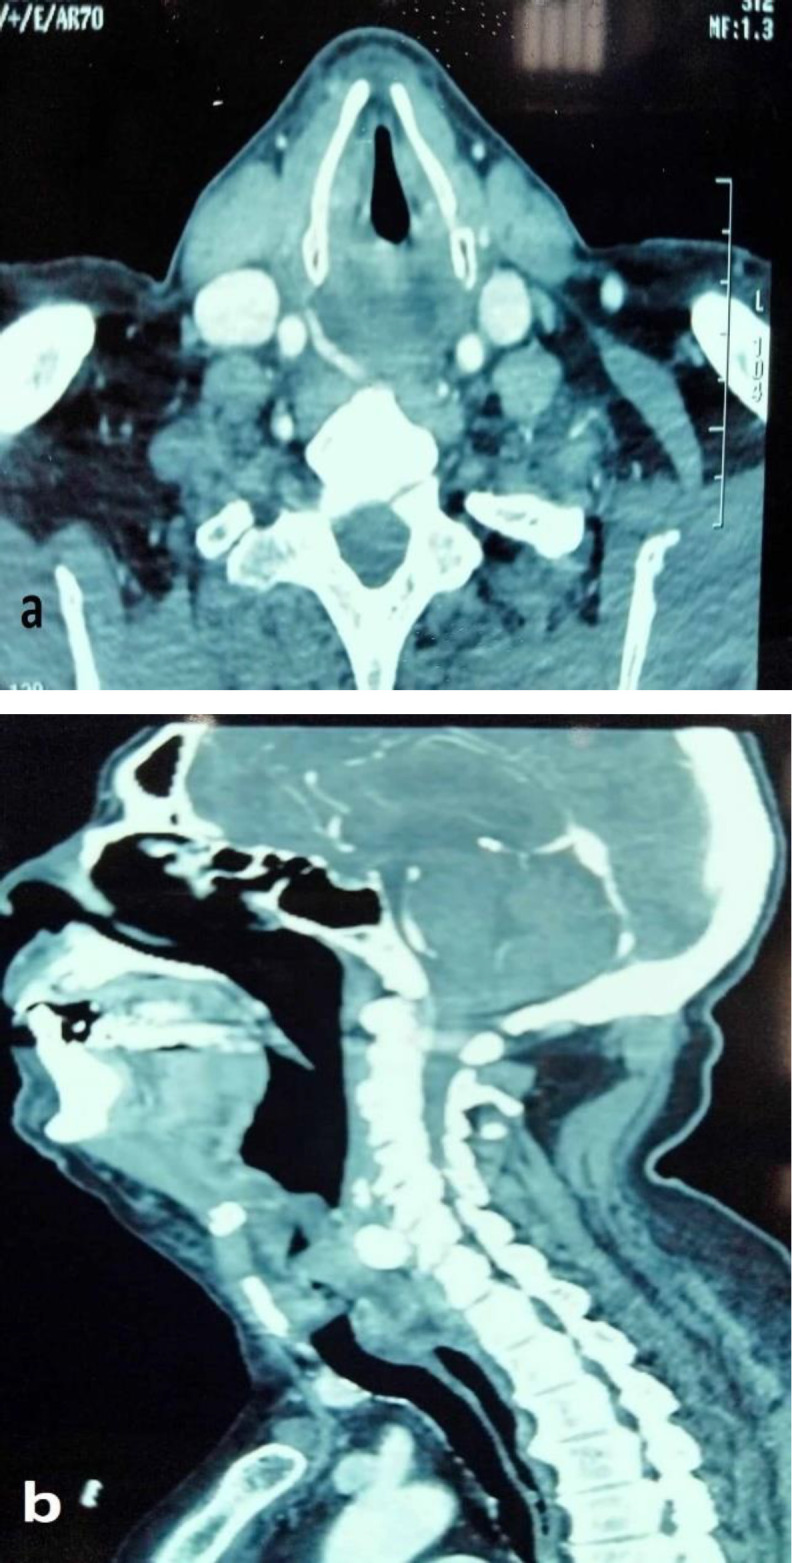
Paryngo-laryngeal soft tissue swelling on axial (a) and coronal (b) CT scan
